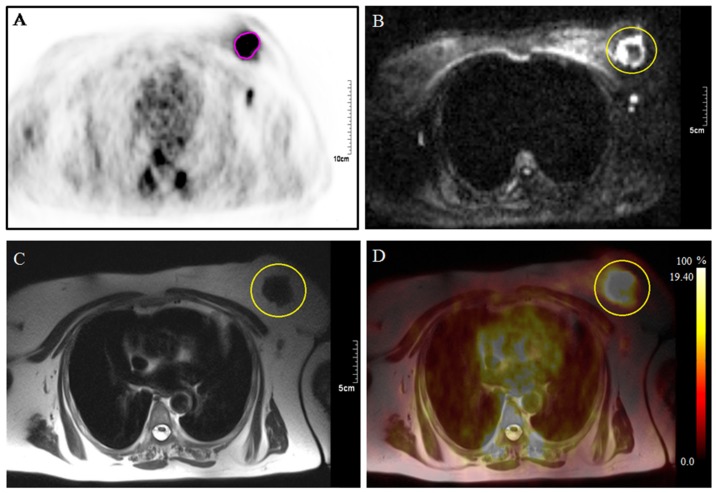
^18^F-FDG-PET/MRI scan Images of a 57-year-old patient with a breast heteroplasia with lymph node and bone metastases. The yellow circle indicates the lesion located on the left breast: (A) PET image showing uptake after 60 minutes of a segmented lesion (pink depicted); (B) Lesion revealed on T2 weighted image acquired on the axial plane; (C) hyperintensity of the lesion on diffusion-weighted magnetic resonance imaging; (D) ^18^F-FDG-PET/MRI fusion image.
